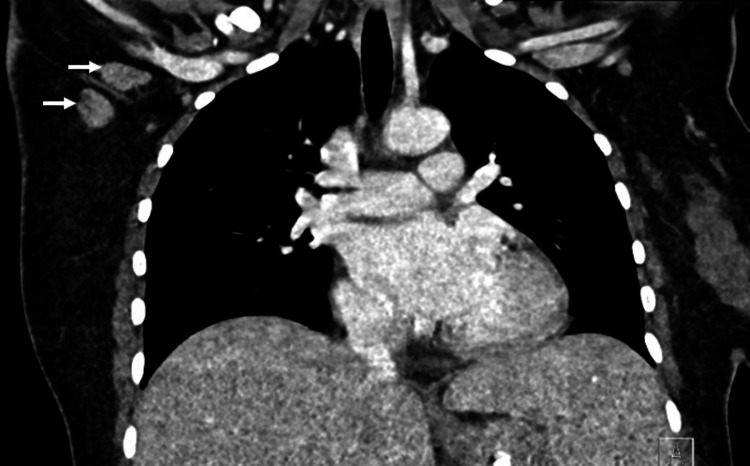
Selected coronal reconstructed image of contrast-enhanced CT chest showing enlarged right side axillary lymph nodes with obliterated fatty hilum (white arrows).
